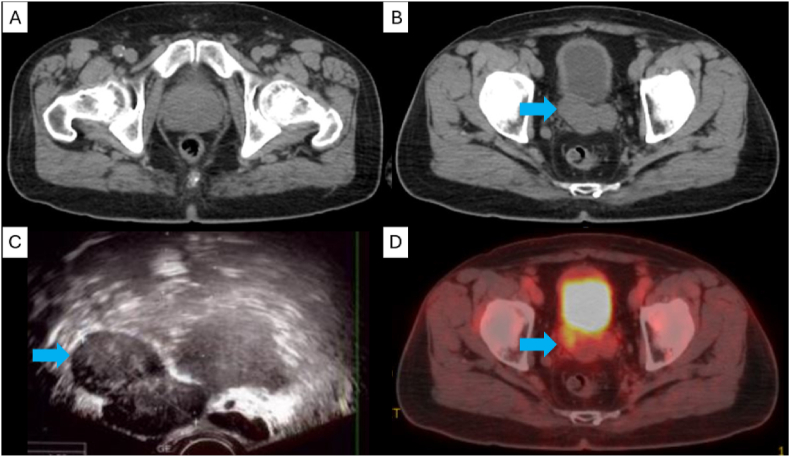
A) Axial CT scan demonstrating benign prostatic hypertrophy measuring 4.2cm × 6.1cm x 5.4cm, without tumor. B) Axial CT scan demonstrating nodular seminal vesicle mass. C) Transrectal ultrasound demonstrating seminal vesicle mass. D) PET-CT demonstrating increased metabolic uptake in the right lateral seminal vesicle.
